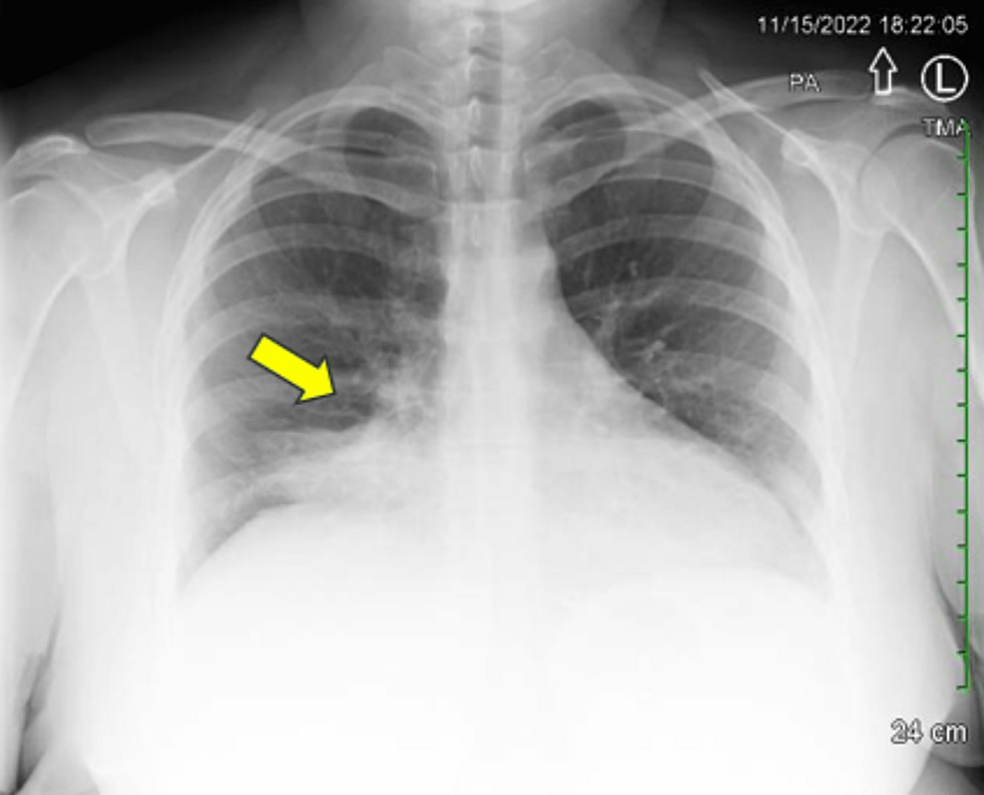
Posterior/anterior chest X-ray from November 2022. The yellow arrow points toward the tumor region.
